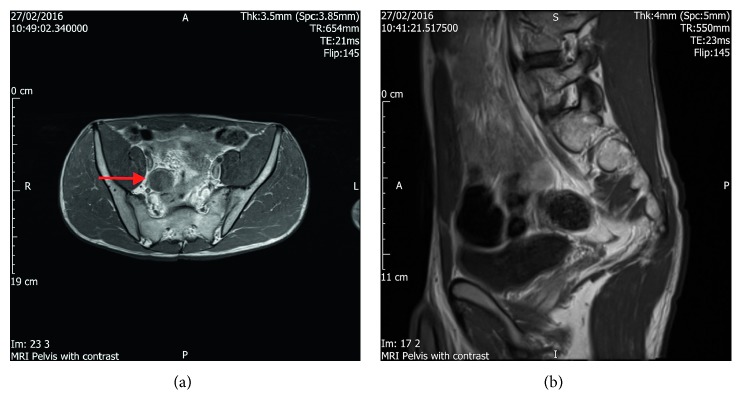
Initial MRI (without contrast) images.
